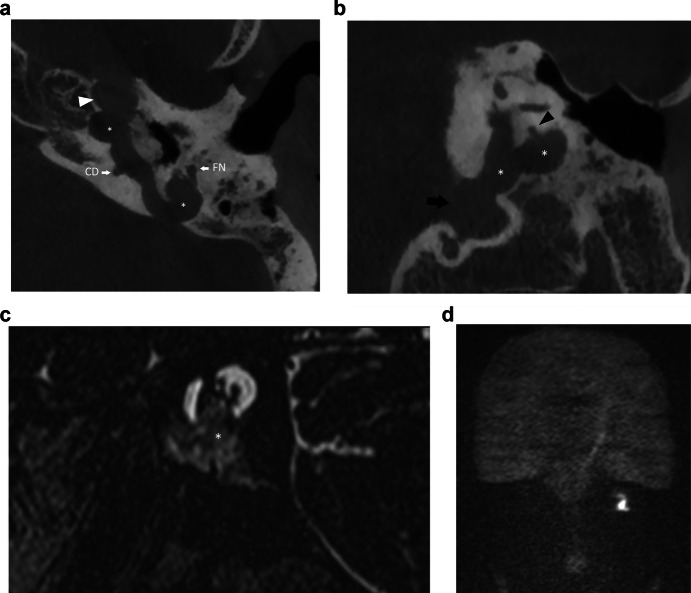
Forty-eight-year-old man with an infralabyrinthine (Class II) CPBC in the left temporal bone. Axial (a) and coronal (b) CBCT images of the left temporal bone show a well-circumscribed C-shaped lytic lesion in the infralabyrinthine region (asterisks in a, b). The lesion has eroded the skull base down to the sigmoid sinus inferiorly (black arrow in b) and the carotid canal anteriorly (white arrowhead in a). Posteriorly there is a dehiscence of the posterior fossa and of the posterior semicircular canal (black arrowhead in b). Sagittal heavily T2-WI (c) displays the growth in the IAC and possible dehiscence to the cochlea (asterisk). There is high DWI signal intensity in the infralabyrinthine petrous bone on non-EP DWI (b1000) (d) compatible with CC. CD, cochlear duct; FN, facial nerve
